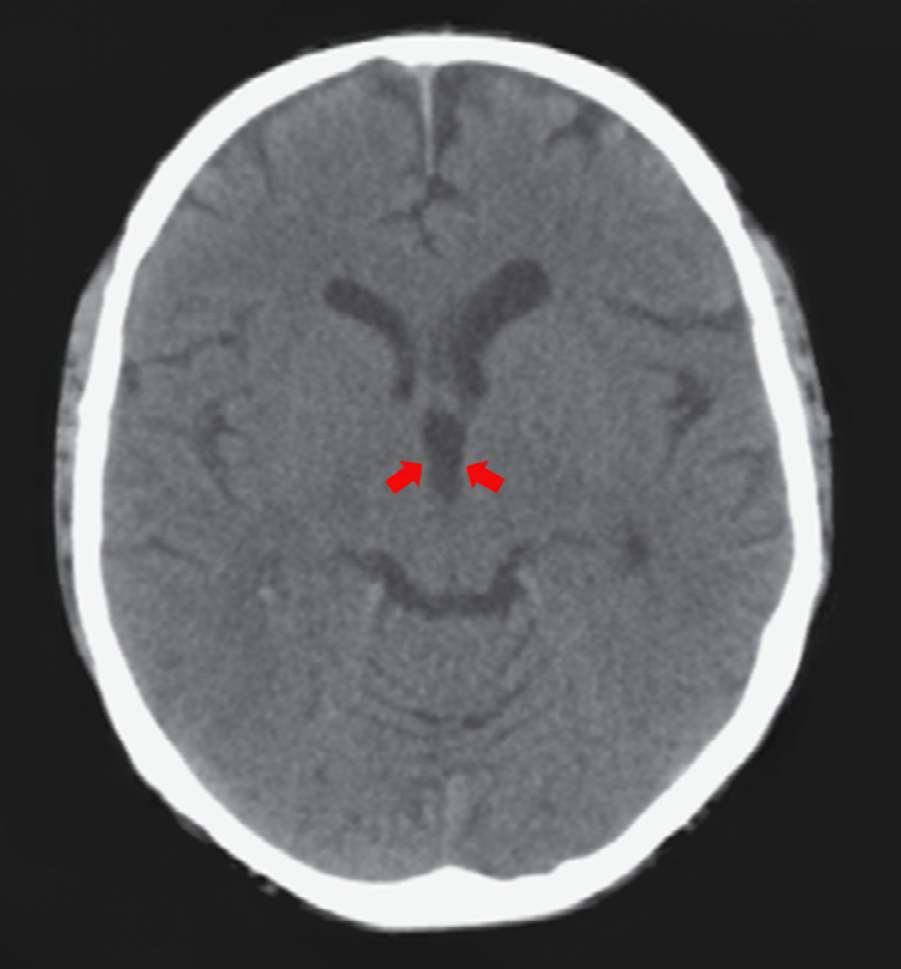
Axial CT image without contrast 7 days postoperatively, showing total cyst excision and reduction of the preoperative hydrocephalus with a completely patent third ventricle (*arrows*).
